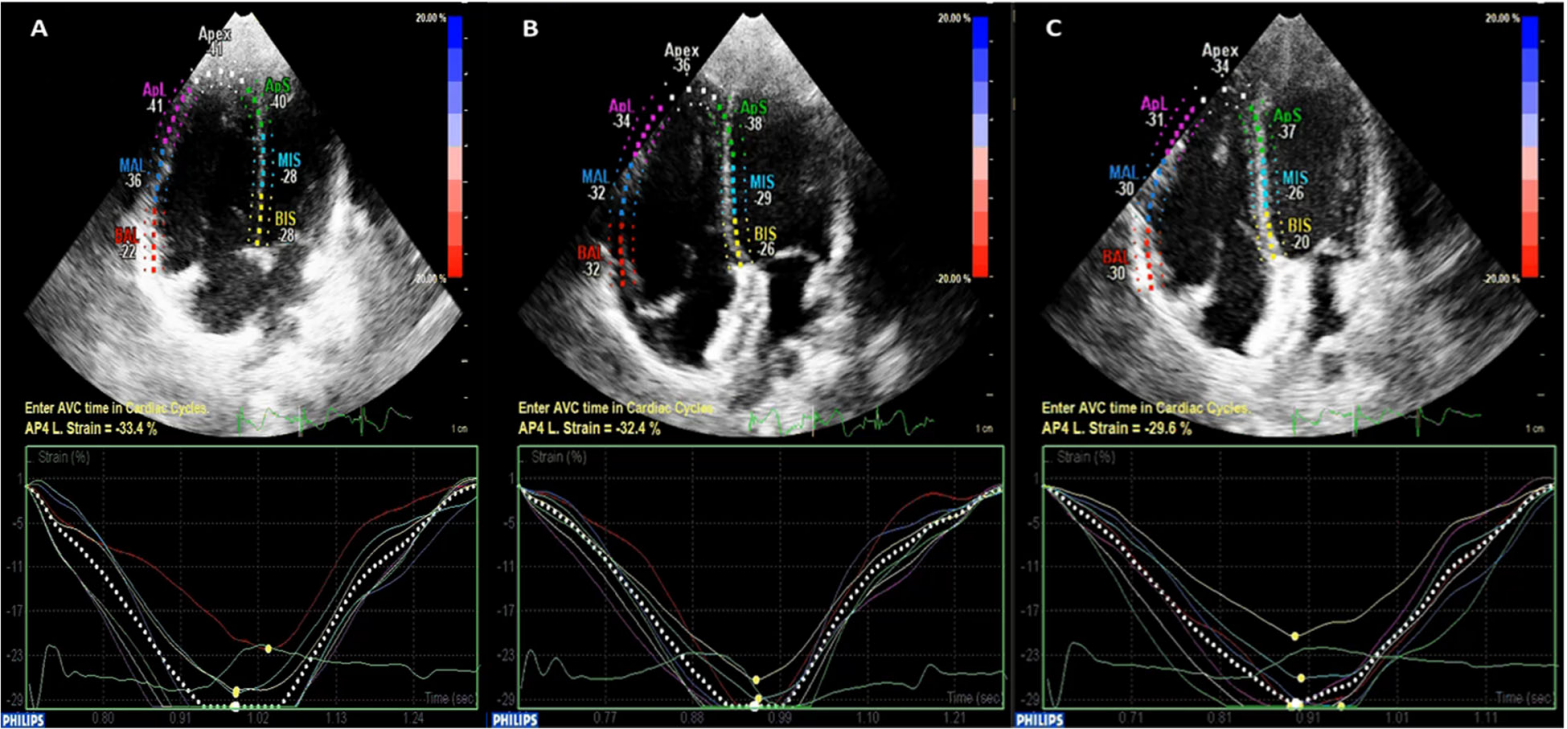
2D-TTE of the RVGLS changes in an ASD case before and after device closure. a Hyperkinetic RV before ASD device closure with RVGLS in apical 4 chambers view (− 33.4%). b Reduction in RVGLS at 24 h post-ASD device closure in the same view (− 32.4%). c Progressive insignificant reduction in RVGLS 1 month post-ASD device closure in the same view (− 29.6%). AP4L, apical 4 chambers longitudinal view; ApL, apical lateral; ApS, apical septum; AVC, aortic valve closure; BAL, basal anterolateral; BIS, basal inferoseptal; MAL, mid-anterolateral; MIS, mid-septum; ASD, atrial septal defect; GLS, global longitudinal strain; RV, right ventricle
